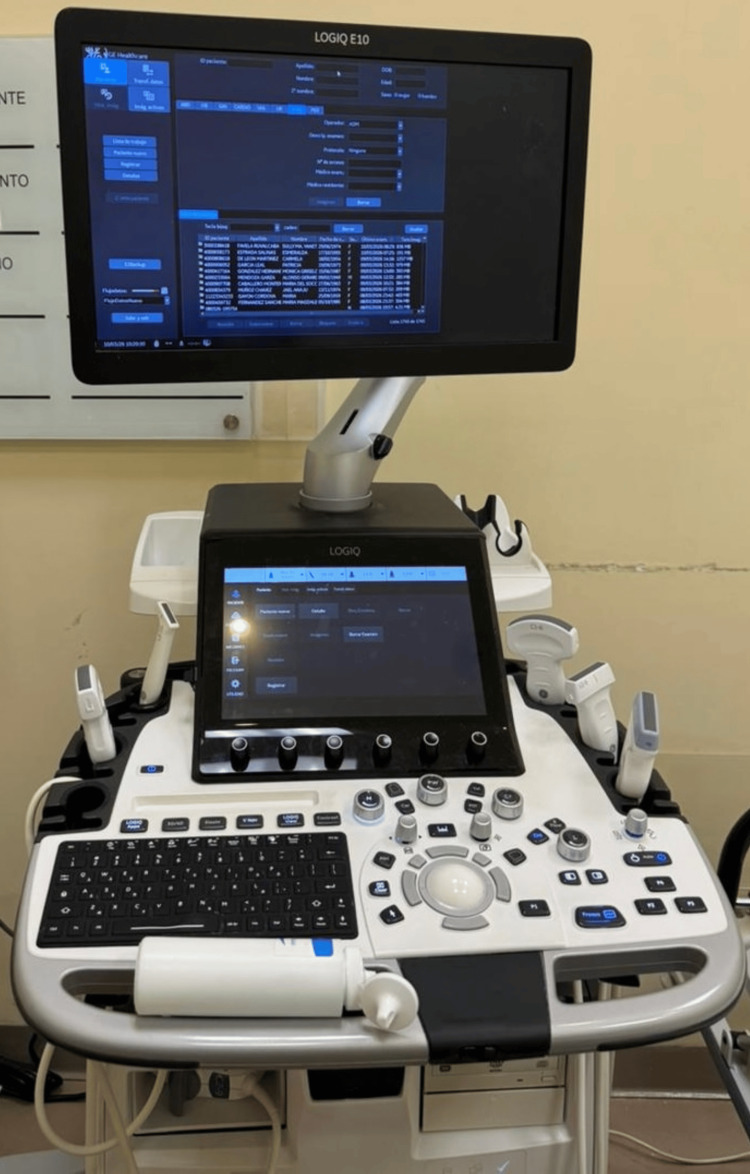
The LOGIQ E10 (GE Healthcare) used for ultrasound image acquisition
